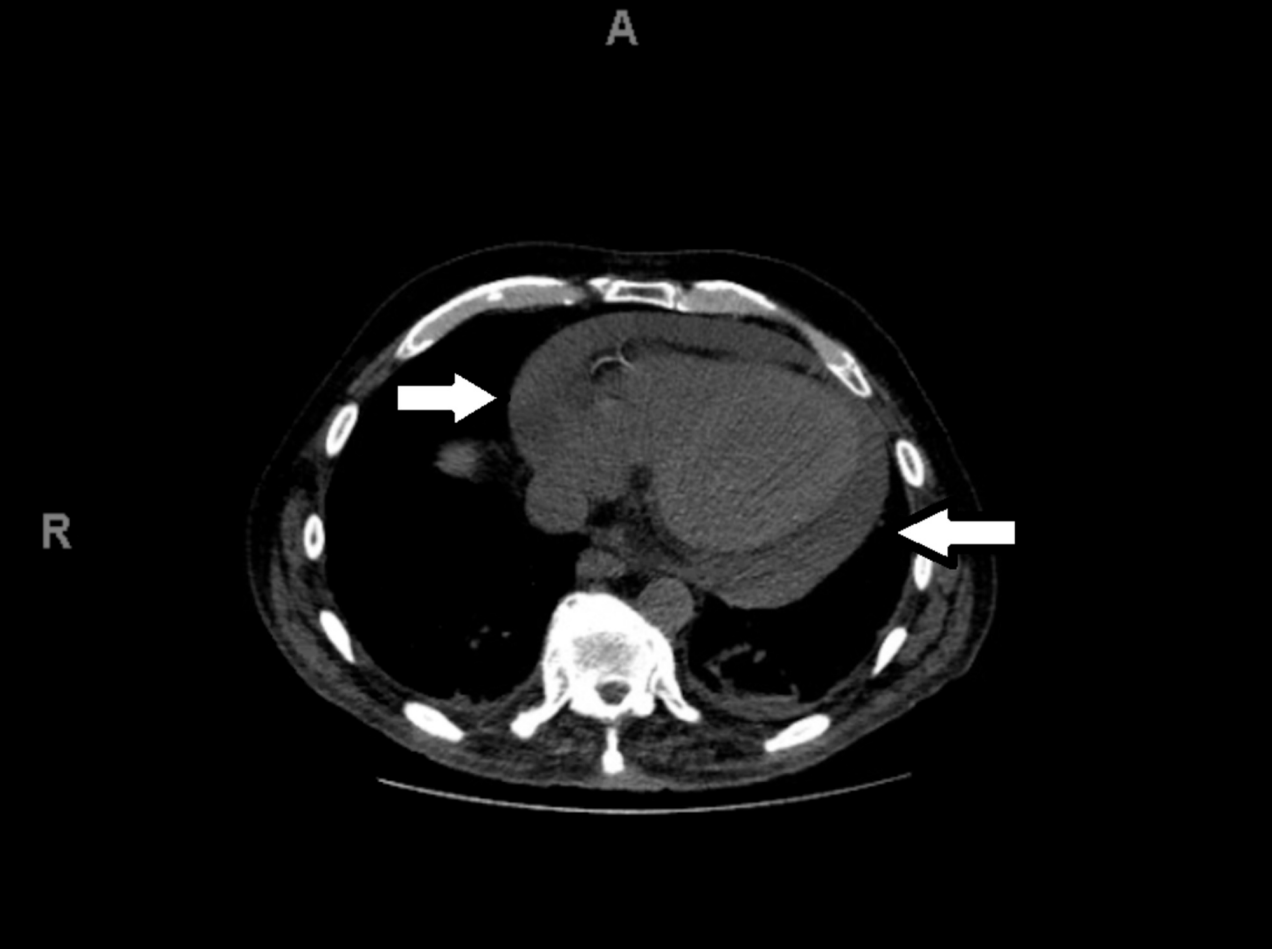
CT scan. Image demonstrating pericardial effusion (white arrows).
